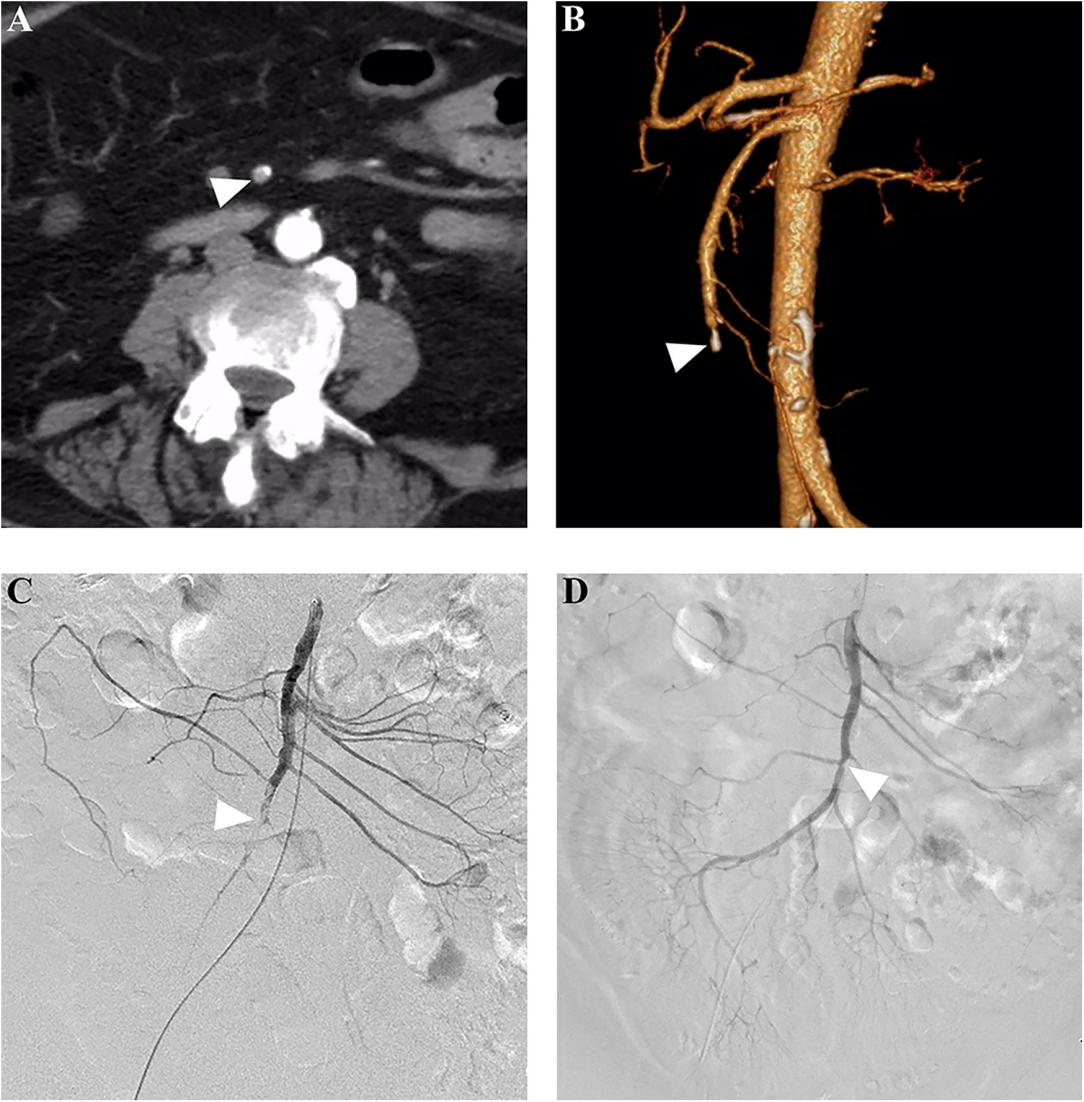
Successful catheter-directed thrombolysis in a patient with acute thrombotic SMA occlusion. (A) A 72-year-old woman presented with acute abdominal pain for 24 h, and subsequent computed tomography angiography revealed a filling defect (arrowhead) in the SMA stem. (B) The three-dimensional reconstruction showed distal SMA stem occlusion and calcification (arrowhead) in the proximal segment of the occluded artery. (C) Pre-procedure angiography showed a filling defect (arrowhead), and an end-hole catheter was placed for local thrombolysis using rt-PA at a rate of 10 mg/24 h. (D) After a thrombolysis duration of 2.0 days, complete revascularization was achieved, and the arrowhead showed a slight stenosis caused by the pre-existing calcification. SMA, superior mesenteric artery.
